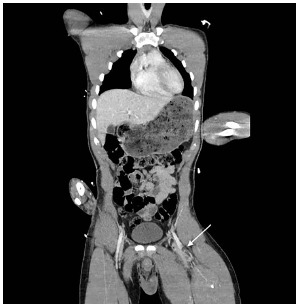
Computed tomography image of the chest, abdomen, and pelvis in coronal view showing fat fluid level within the left common femoral vein (arrow).
